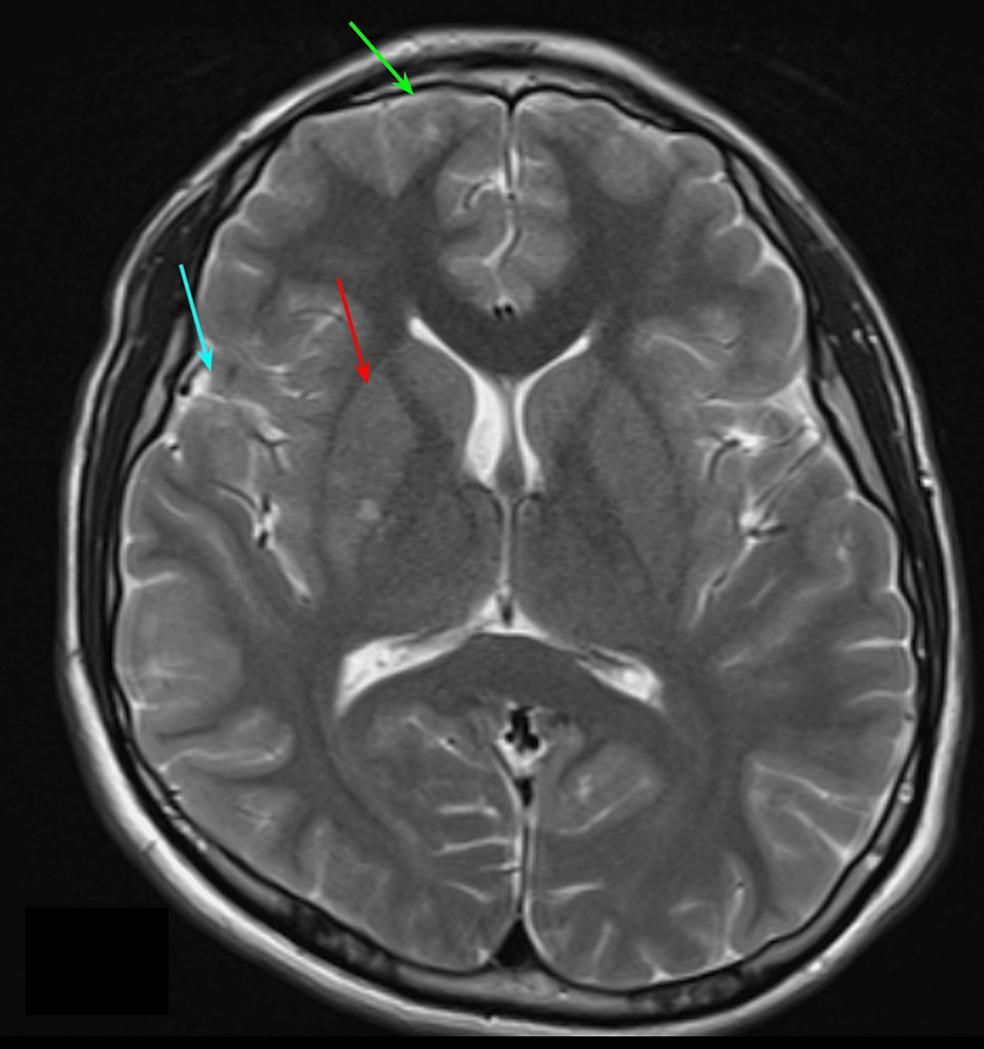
First brain MRI T2 image Hyperacute ischemic vascular disease in the right middle frontal (green arrow) and temporal gyri (blue arrow) with ipsilateral lentiform nucleus involvement (red arrow).
